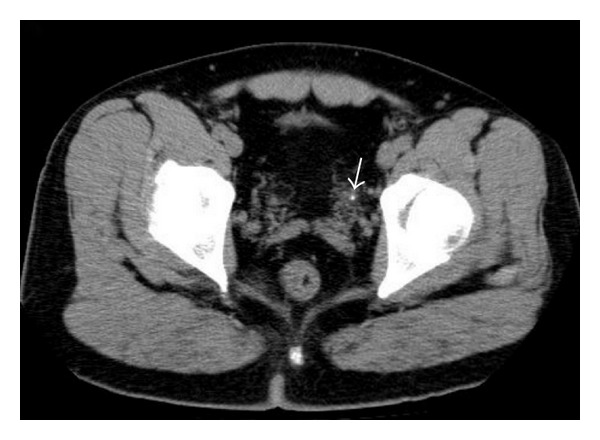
CT showing a small ureteral stone located at the ureterovesical junction area (arrow).
